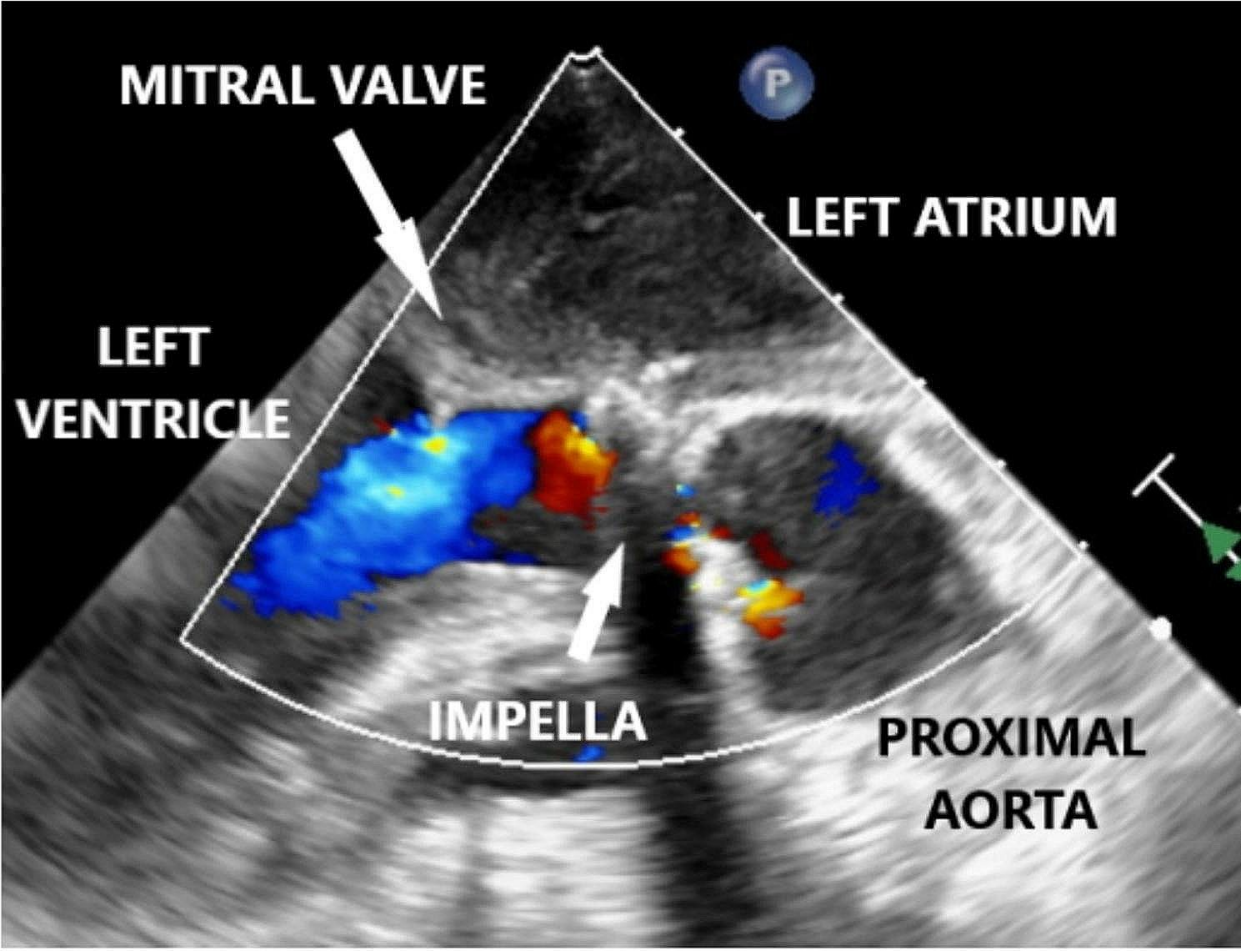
Transesophageal echocardiogram, midesophageal aortic valve long-axis view showing the left atrium with spontaneous echo contrast, a thickened, non-functional mitral valve, the left ventricle with an aortic regurgitant jet, the Impella as it passes through the aortic valve with a large shadowing artifact below it, and the proximal ascending aorta
